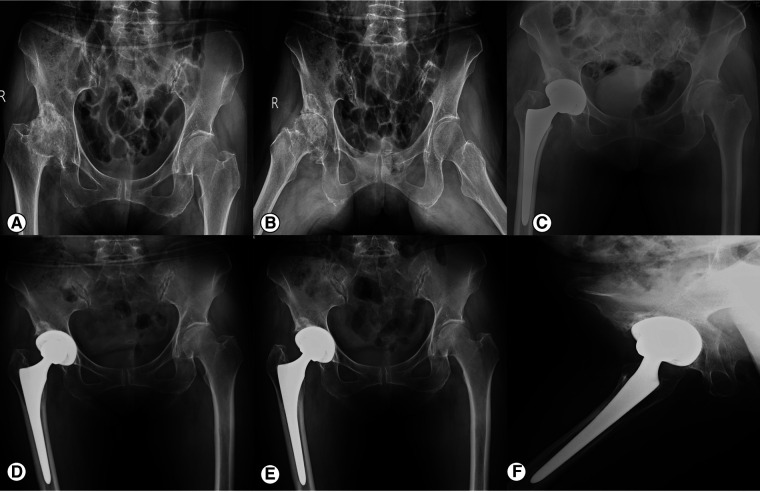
X-ray of a 61-year-old female DDH patient before and after THA. (A, B) The preoperative anteroposterior view of the pelvis and frog-leg X-ray; (C) The anteroposterior view of the pelvis on the first day after operation. The hip rotation center on the operated side has been restored, and the acetabular cup has a well anteversion angle and abduction angle. The femoral stem is implanted in the neutral position. (D) Anteroposterior X-ray of the pelvis 12 months after operation show that the prosthesis is well fixed. (E, F) The anteroposterior view of the pelvis and oblique view of the right hip at 48 months postoperatively. The prosthesis is well fixed and there is no lucent line between the acetabular prosthesis and the host bone.
